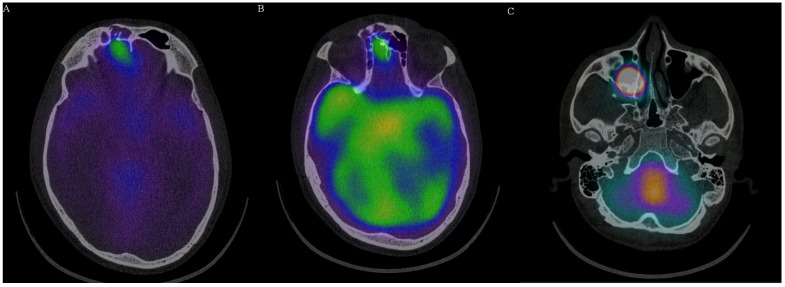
SPECT scanning: (A) axial image of increased radioisotope activity over the right frontal sinus with bone defect; (B) axial image of increased radioisotope activity over the anterior part cribriform plate with bone defect; (C) radioisotope activity in the right maxillary sinus. (Images provided with the courtesy of the Institute on Nuclear and Molecular Medicine, Kosice.).
